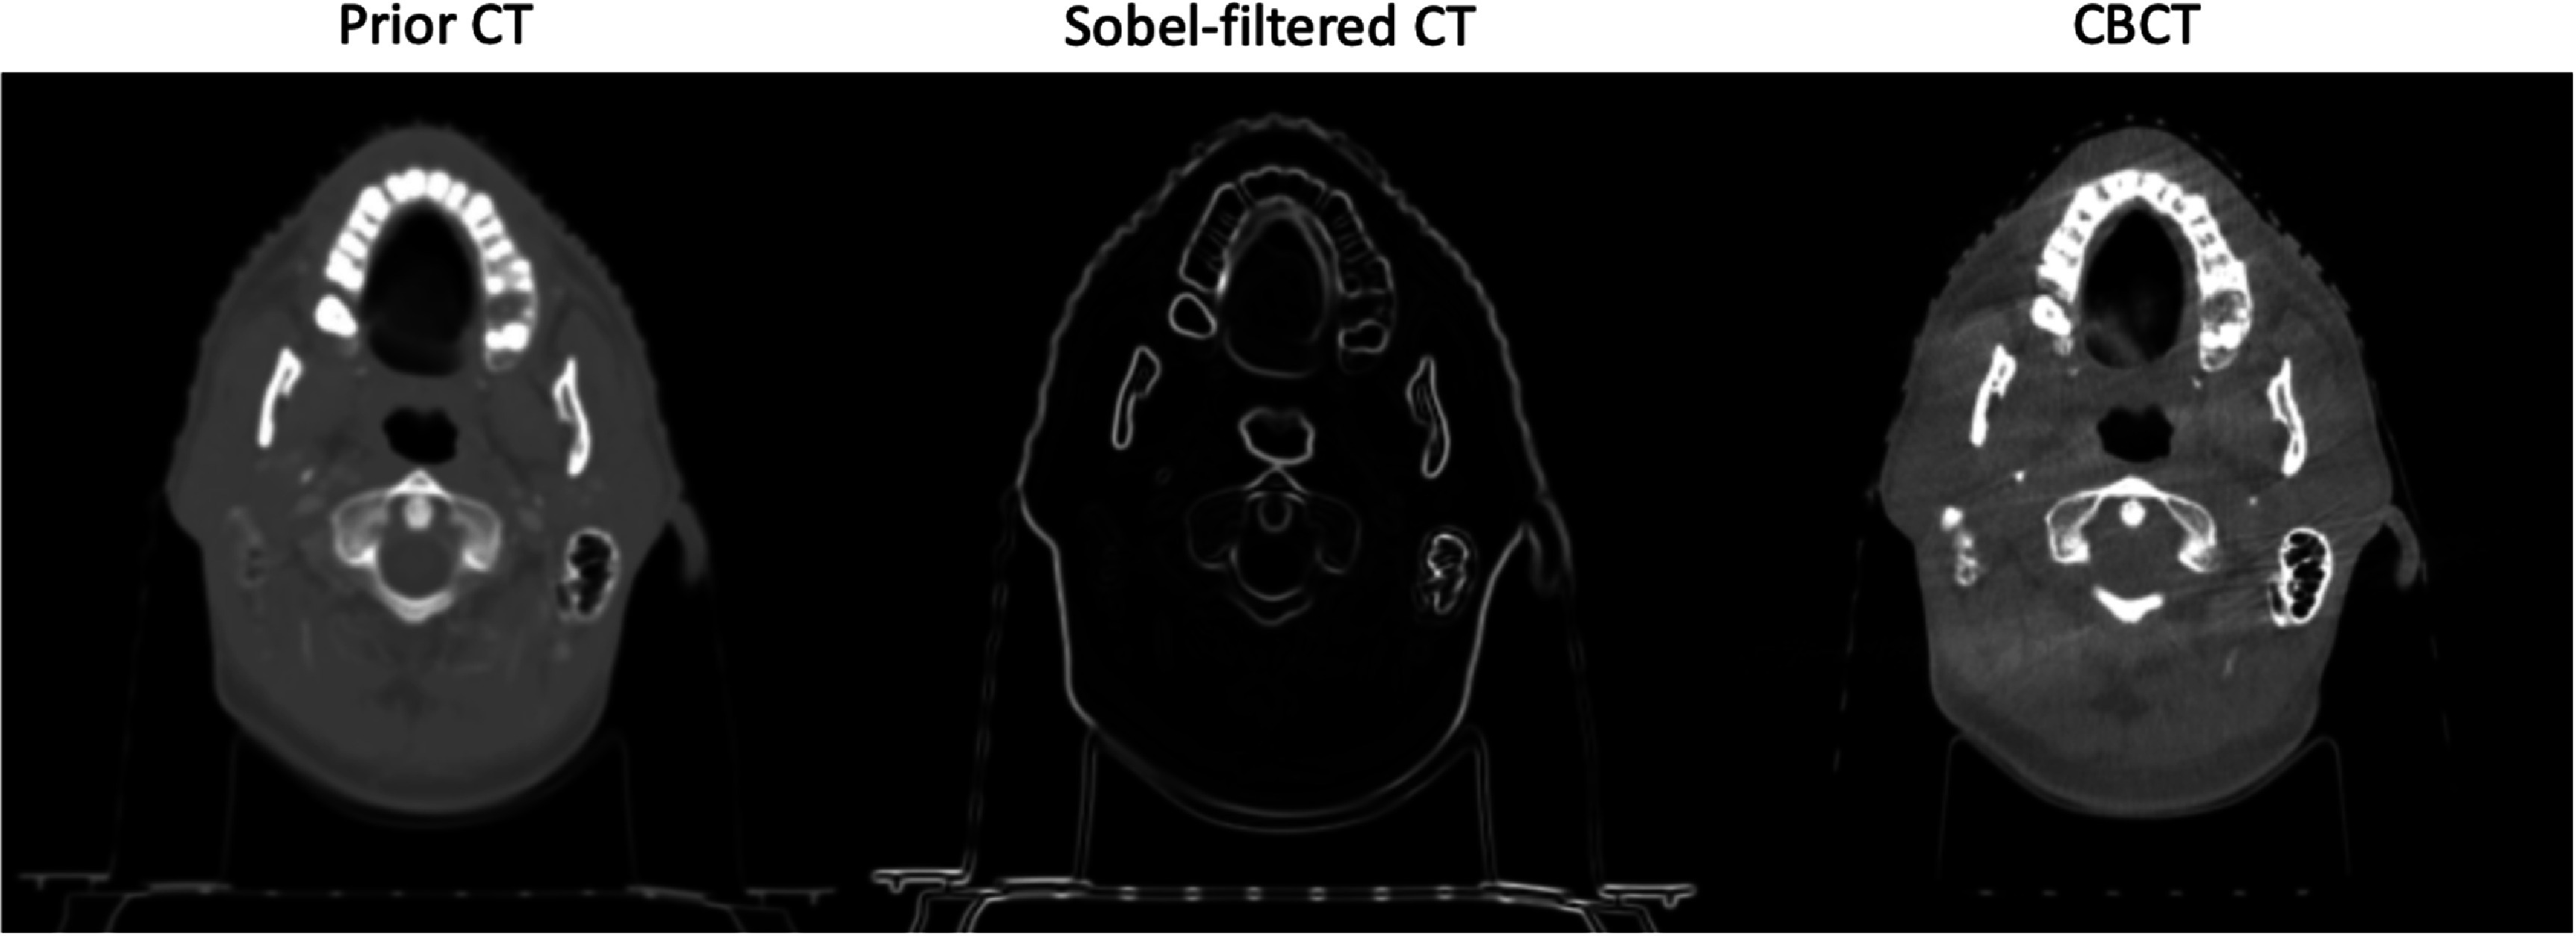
Samples from the head and neck dataset: the prior CT scan (left), the extracted high-frequency information of the prior CT scan (middle), and the CBCT scan (right) of the same patient.
